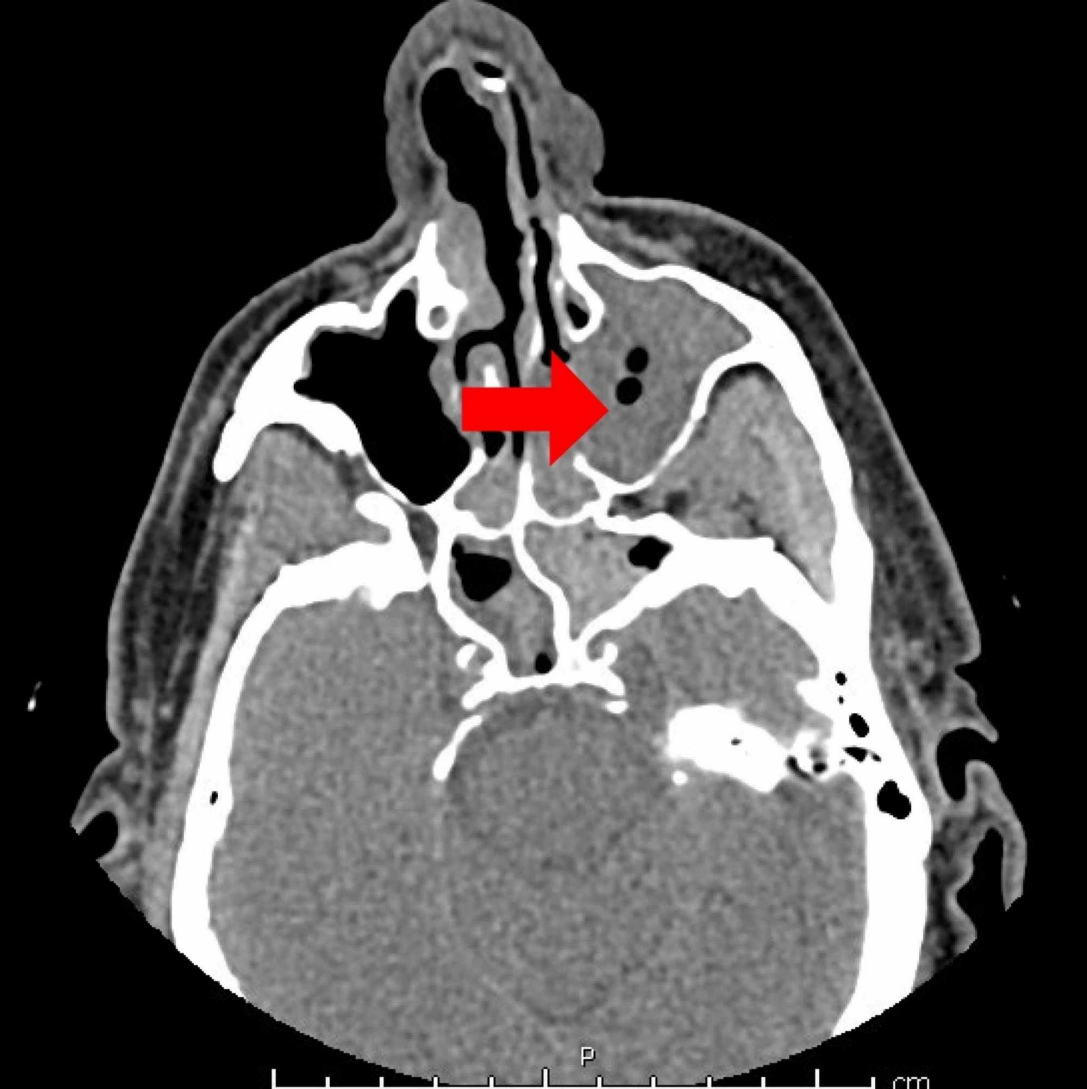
CT maxillofacial without contrast. CT scan maxillofacial without contrast showing severe opacification of left maxillary sinus as pointed by the red arrow
